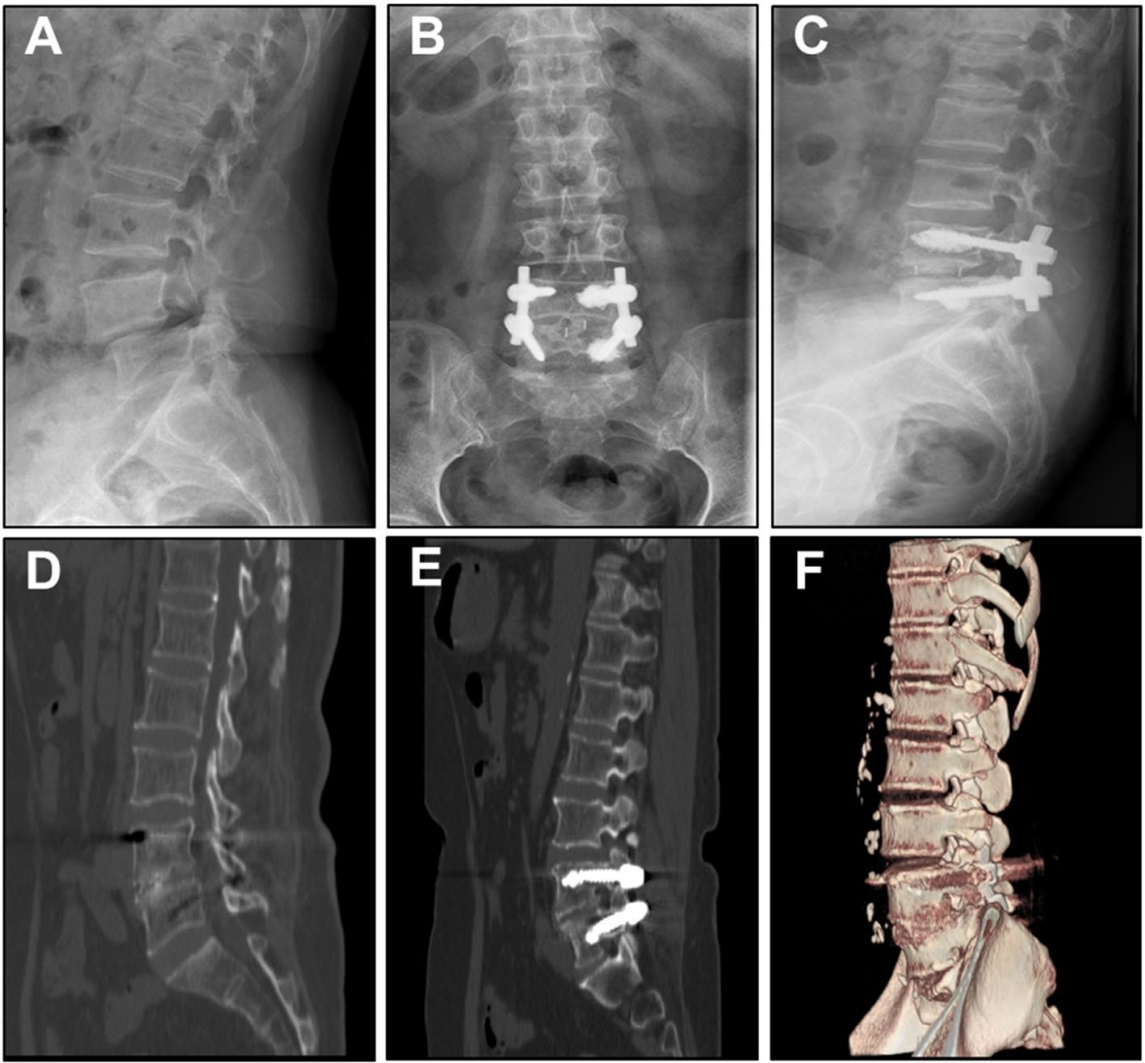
A 59 years old female, BMD -2.7SD, treated with CICPS fixation by PMMA augmentation. A Preoperative radiographs showed L4 vertebral body was of level II forward slip, spondylolysis type. B and C No bone cement leakage was observed one week after surgery. D CT results indicated successful fusion six months after surgery that continuous bone callus passed in the intervertebral space of fusion. E and F 56 months after the operation, CT showed good intervertebral fusion, no loosening or pulling out of screw was observed
